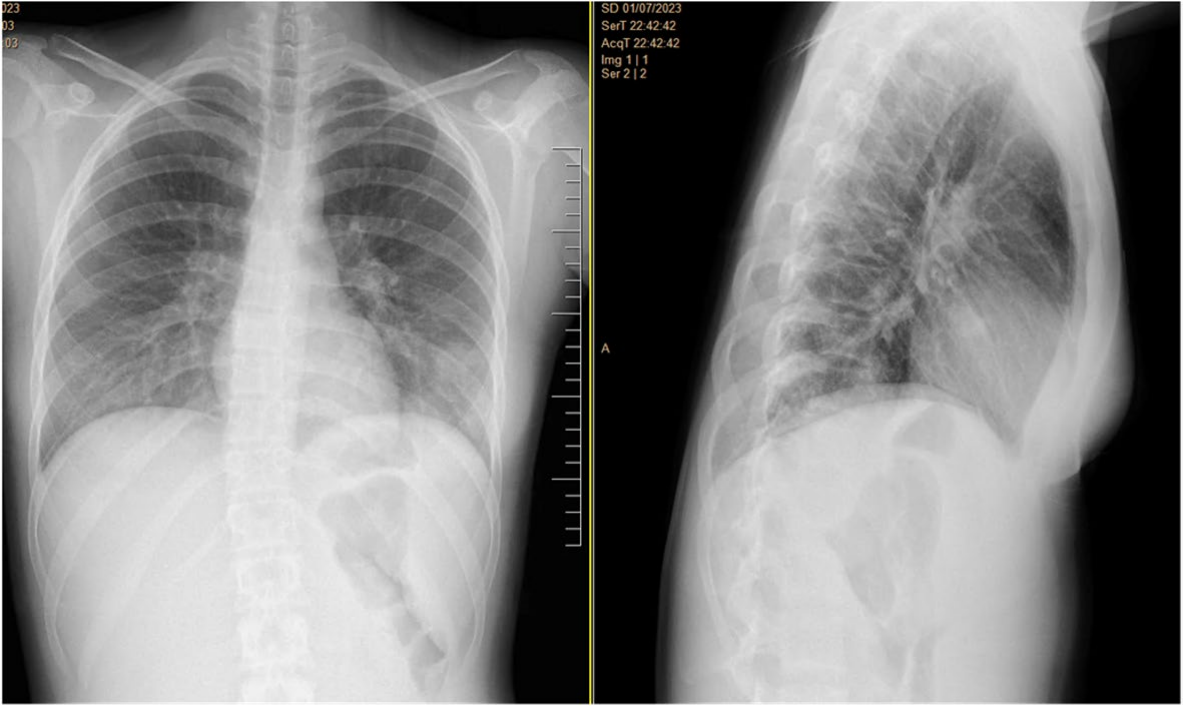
Chest X-Ray: consolidation focus in the left lower lobe of the lung
